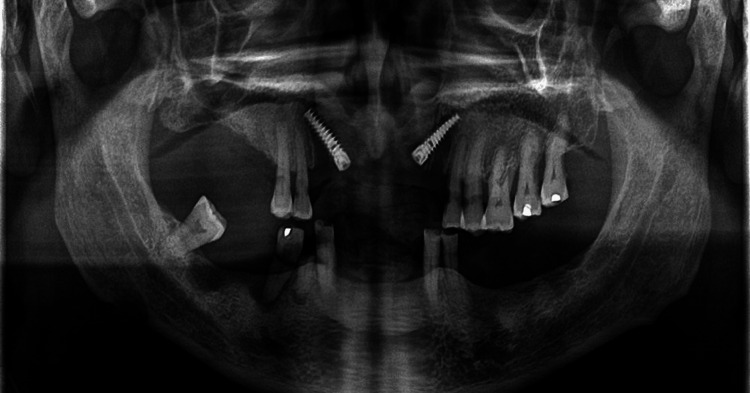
Post-operative orthopantomograph (Case 2)
